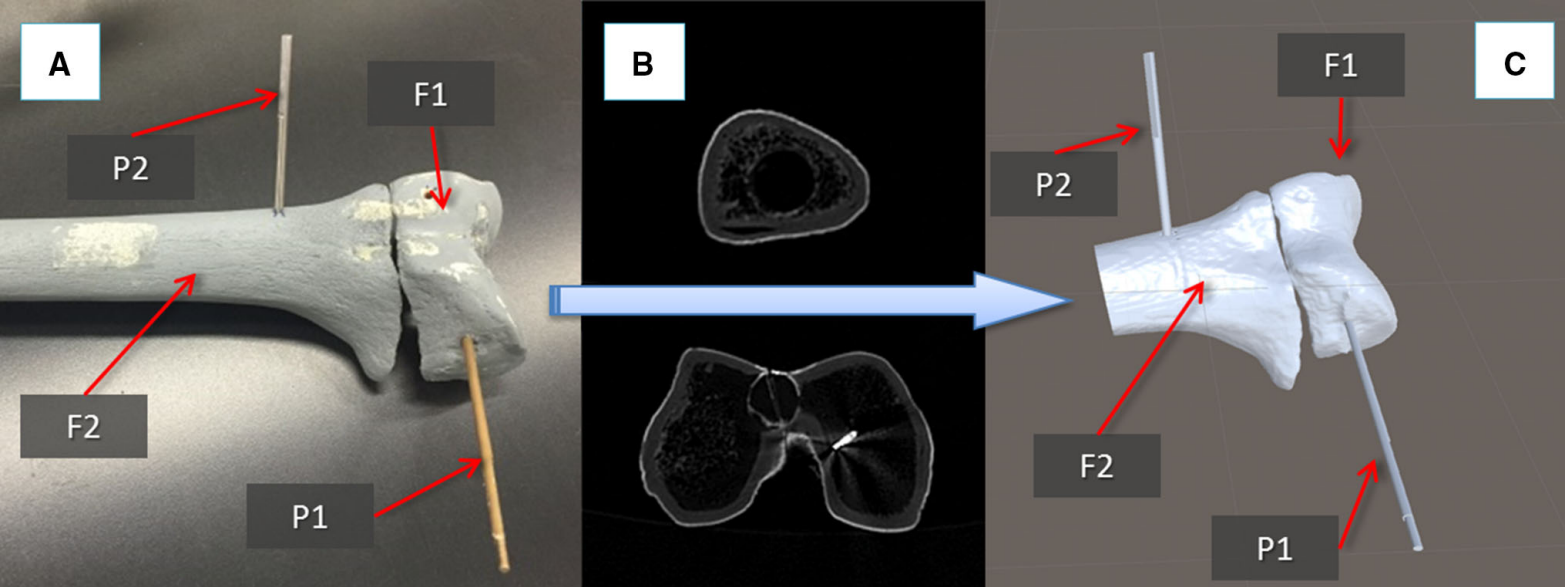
Fractured femur model with orthopaedic pins inserted (a), relative CT images (b), and 3D models (c)
